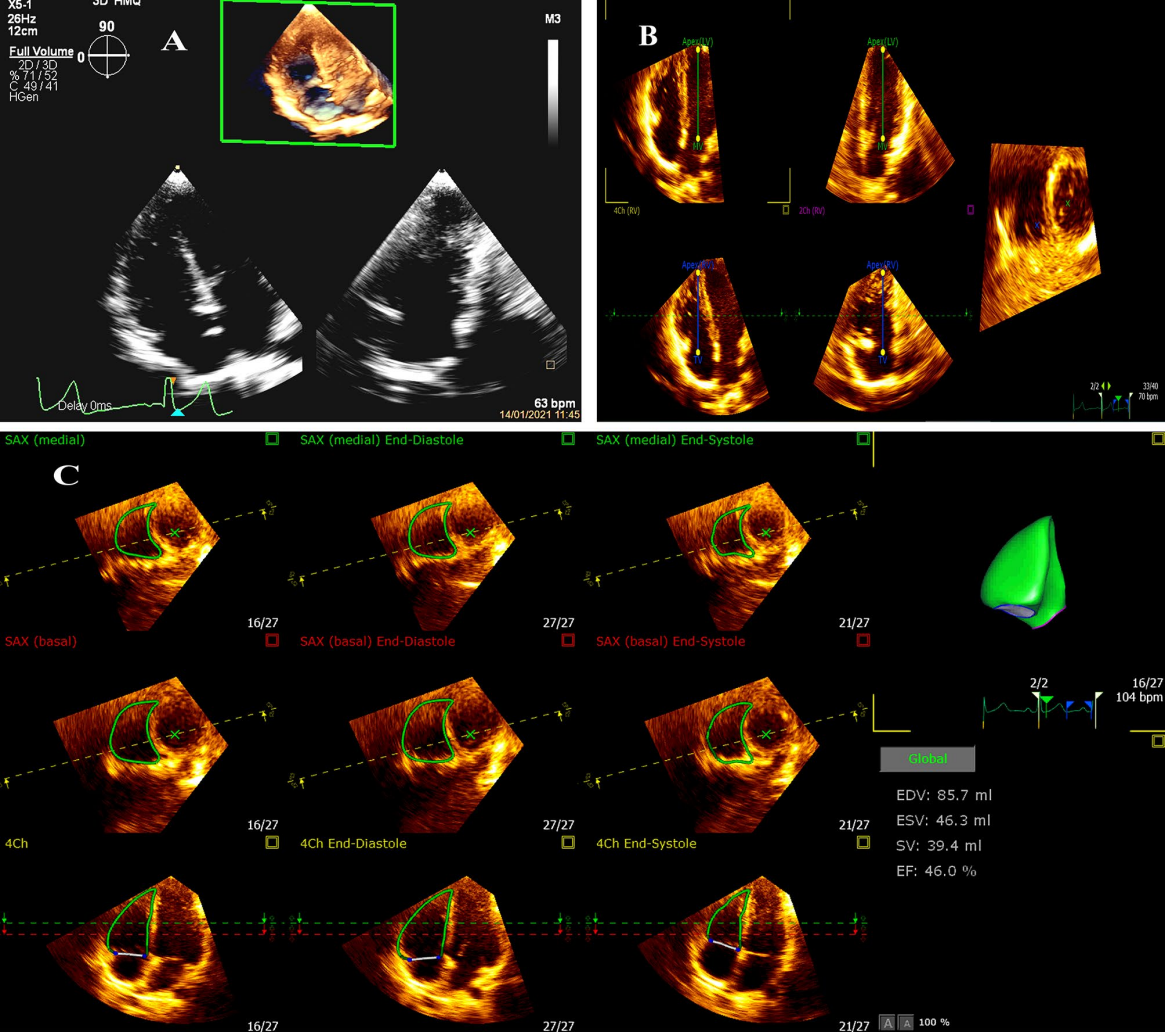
The fully automated right ventricular (RV) functional assessment. A The RV focus three-dimensional echocardiography (3DE) full-volume data set acquisition. B The software adjusts the five landmarks automatically, which are adjusted as needed. C Automatic tracking of the RV border at the end diastole and end systole and the results of SLE patient showing 3D-derived RV EF 46%
